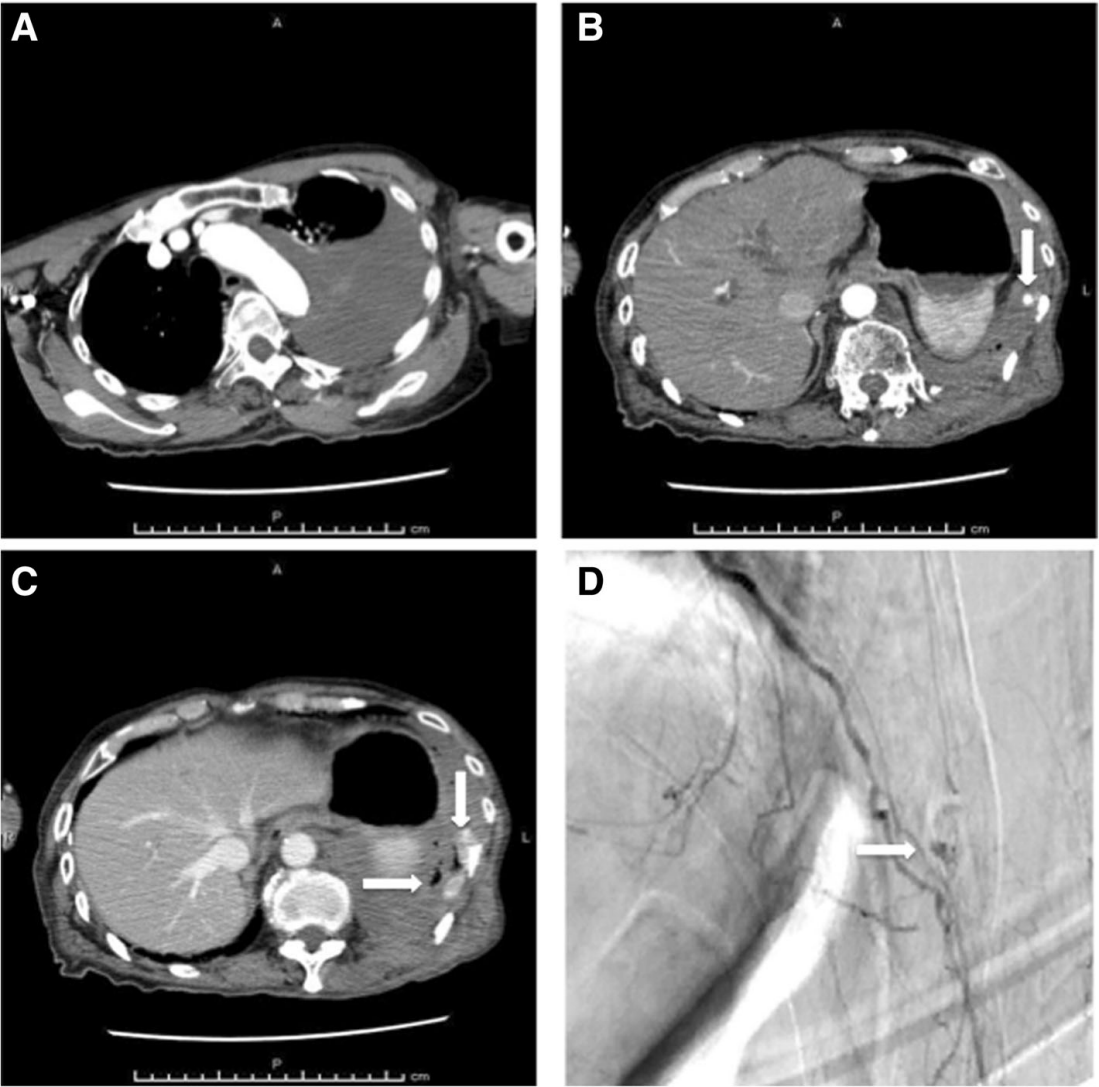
Contrast-enhanced computed tomography and angiography findings. Contrast-enhanced computed tomography (a) shows increased hemothorax compared with that shown in Fig. 1b. Early-phase and late-phase contrast-enhanced computed tomography images show extravasation of intercostal artery (*arrow*; b) and increased area of extravasation (*arrows*; c), respectively, near tenth rib. Angiography (d) shows extravasation (*arrow*)
